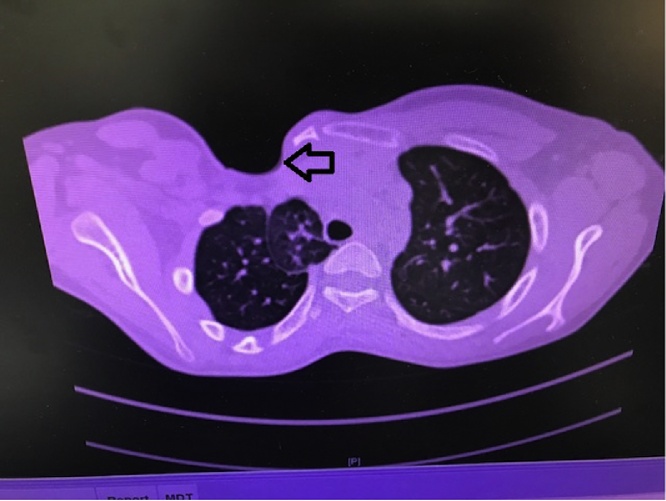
Preoperative CT Scan showing the skin over the pleura with no muscles across the defect (the arrow points to the defect).
